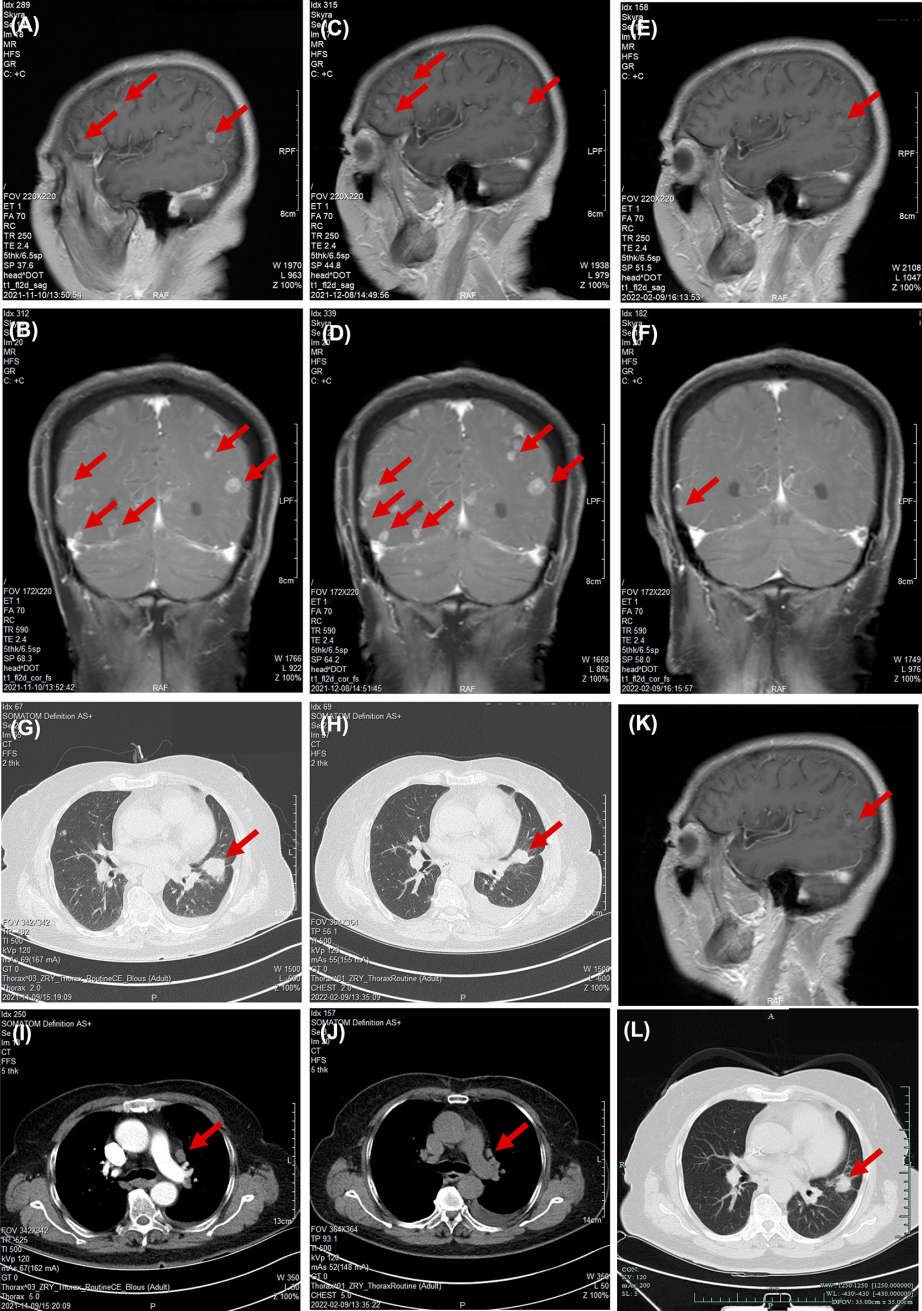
Responses of EGFR TKIs as third- and fourth-line therapy. Chest scanning CT before and after EGFR TKI treatment: (A, B) Brain MRI before gefitinib on November 10, 2021. (C, D) Brain MRI after gefitinib on December 8, 2021. (E, F) Brain MRI after almonertinib on February 9, 2022. (G, H) Chest CT scan before gefitinib on November 10, 2021. (I, J) Chest CT scan after almonertinib on February 9, 2022. (K, L) Chest CT and brain MRI 4 months post-almonertinib on April 14, 2022. Significant abnormal findings noted (*arrow*).
